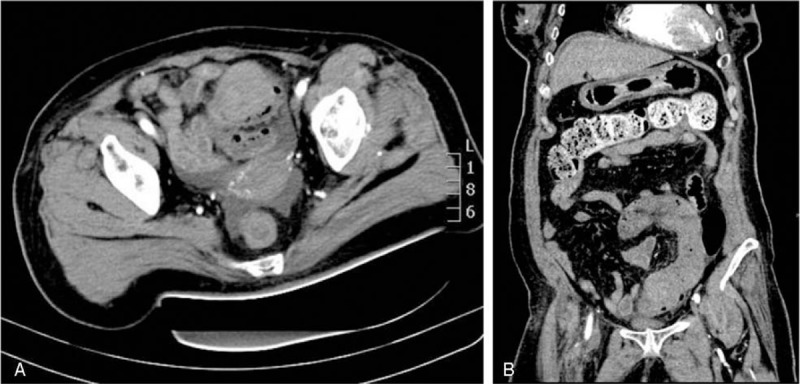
(A, B) Plain computed tomography showed hyperdense lesion in the intramural aspect of the sigmoid colon.
